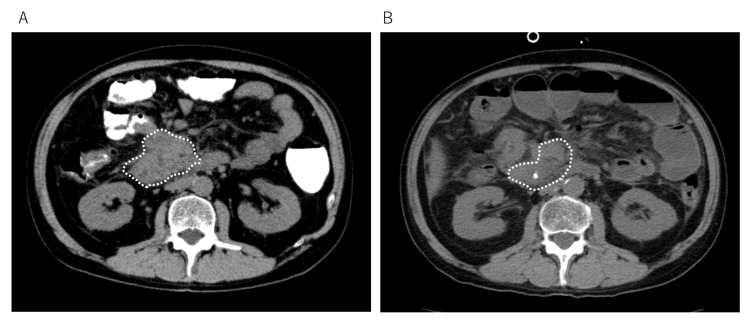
Change in cancer size before and after immunotherapy Abdominal CT findings before (A) and after (B) immunotherapy with WT1-DC and α-Galcer-DC. The dotted line indicates the small intestine cancer, which shrank significantly after three months of treatment. WT1-DC, Wilms' tumor 1-dendritic cell vaccine; α-Galcer-DC, α-galactosylceramide-dendritic cell vaccine
